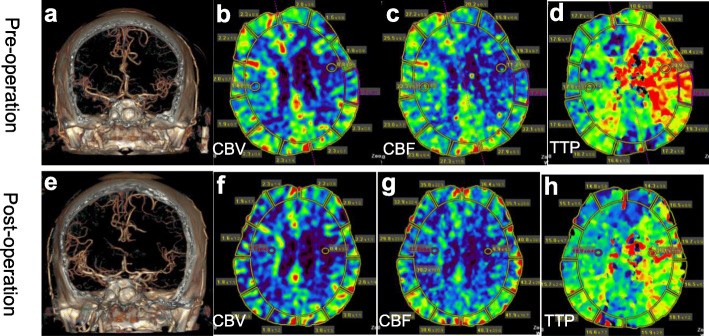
a and e CTA before and 24 hours after stenting show the intracranial vessels are similar. b, cand d CTP before operation. f, g and h CTP 24 hours after operation
